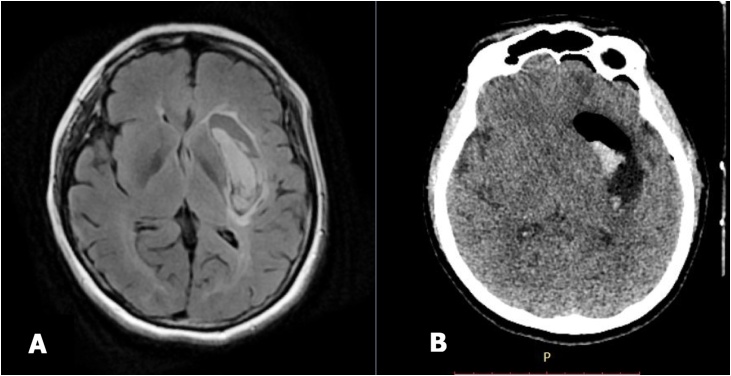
A. Pre-operative MRI a few hour after onset, and B. Post-operative CT scan after stereotactic surgery immediately.
